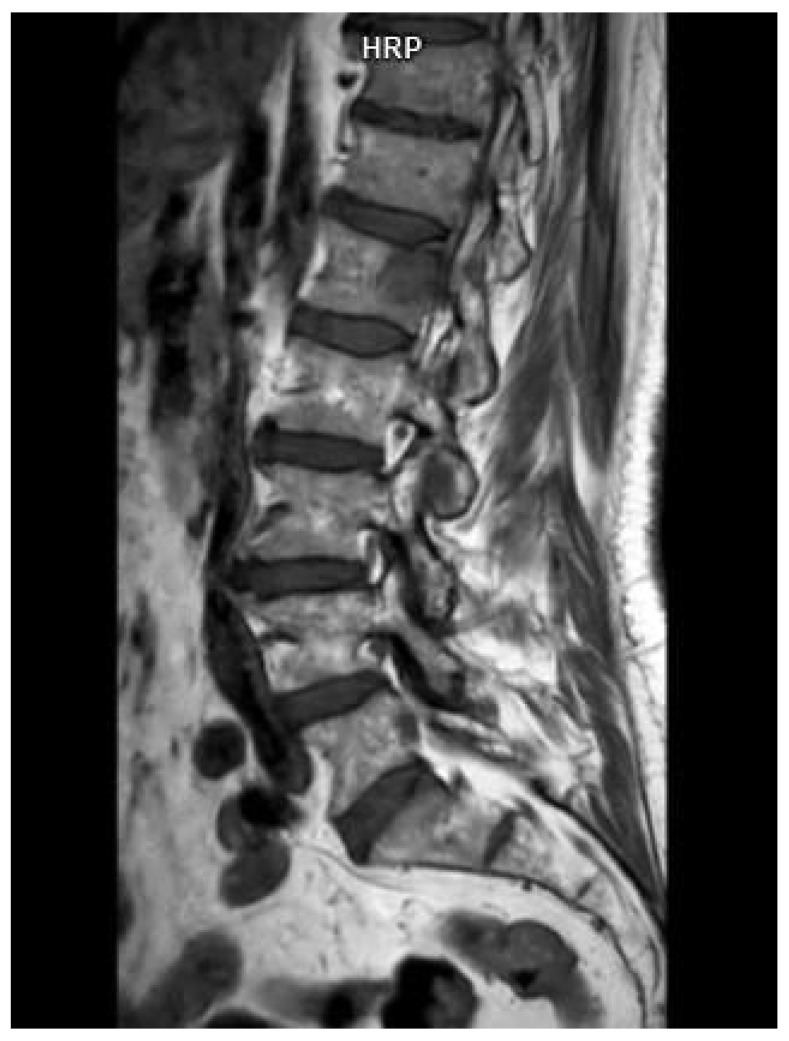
Sagittal T1-weighted image of a spine demonstrating a focal lesion in a 73-year-old man with multiple myeloma diagnosis. Source: Radiological Diagnostics Center, The National Institute of Medicine of the Ministry of Interior and Administration, Warsaw, Poland.
